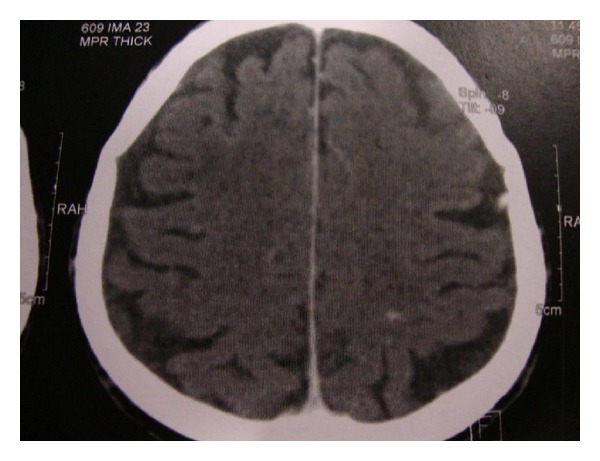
Axial MRI of the brain following 3 months of anti-TB therapy demonstrating an important resolution of the previous brain lesions.
